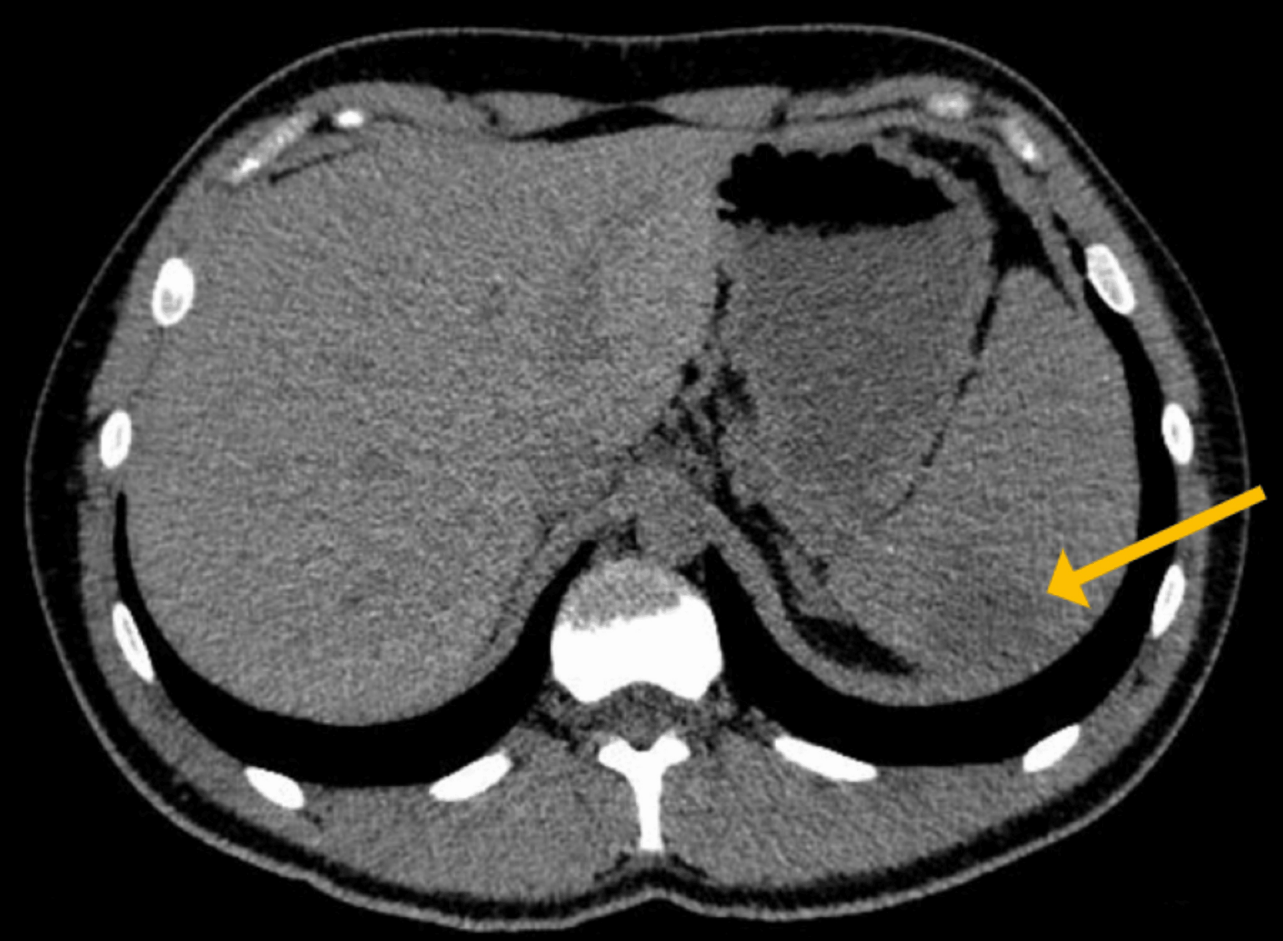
Abdominal CT scan without contrast showing splenomegaly (15.6 cm in craniocaudal dimensions) with a hypodensity at the posterior part of the upper pole of the spleen measuring 4 x 3 cm, corresponding to a splenic infarct.
